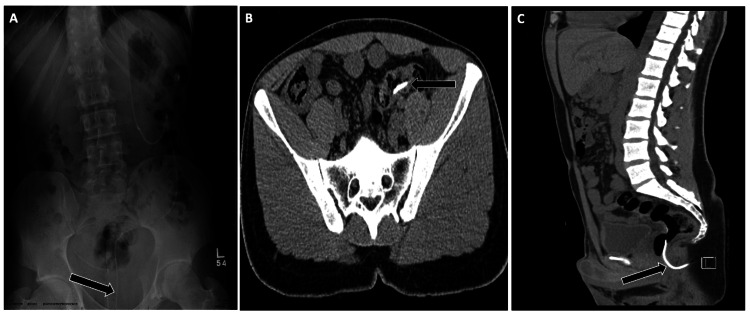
Distal Migration and Extrusion of a Ventriculoperitoneal (VP) Shunt Through the Anus Imaging of a Migrated VP Shunt with Distal Catheter Extrusion (A) Abdominal radiograph demonstrates the VP shunt catheter (arrow) projecting over the region of the descending/sigmoid colon and rectum, with the distal tip extending beyond the field of view. (B) Axial non-contrast CT image of the abdomen shows the catheter coursing through the sigmoid colon lumen (arrow). (C) Sagittal non-contrast CT of the abdomen and pelvis confirms the distal shunt tip traversing the rectum and extruding through the anus (arrow). This rare but serious complication carries a high risk of infection and requires urgent surgical management.
